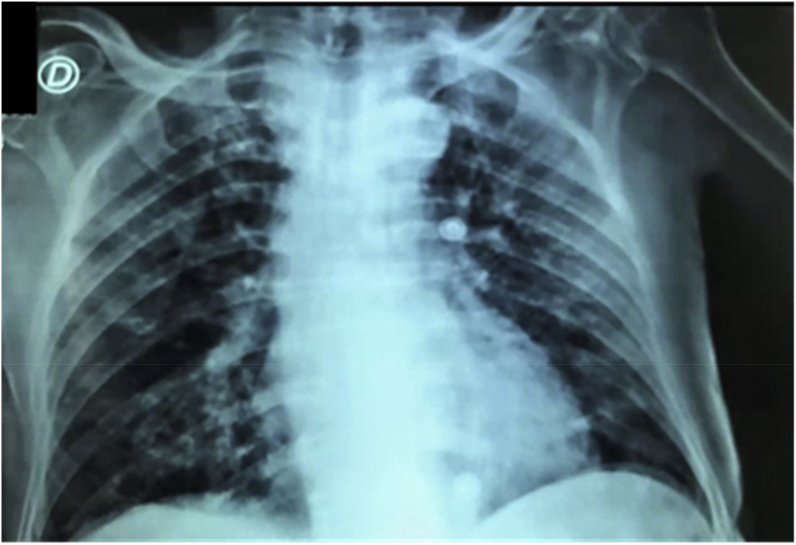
Chest X-ray evidenced bilateral heterogeneous infiltrates on day 26 of hospitalization: picture from the COVID-19 associated pulmonary aspergillosis (CAPA) described on this report.
